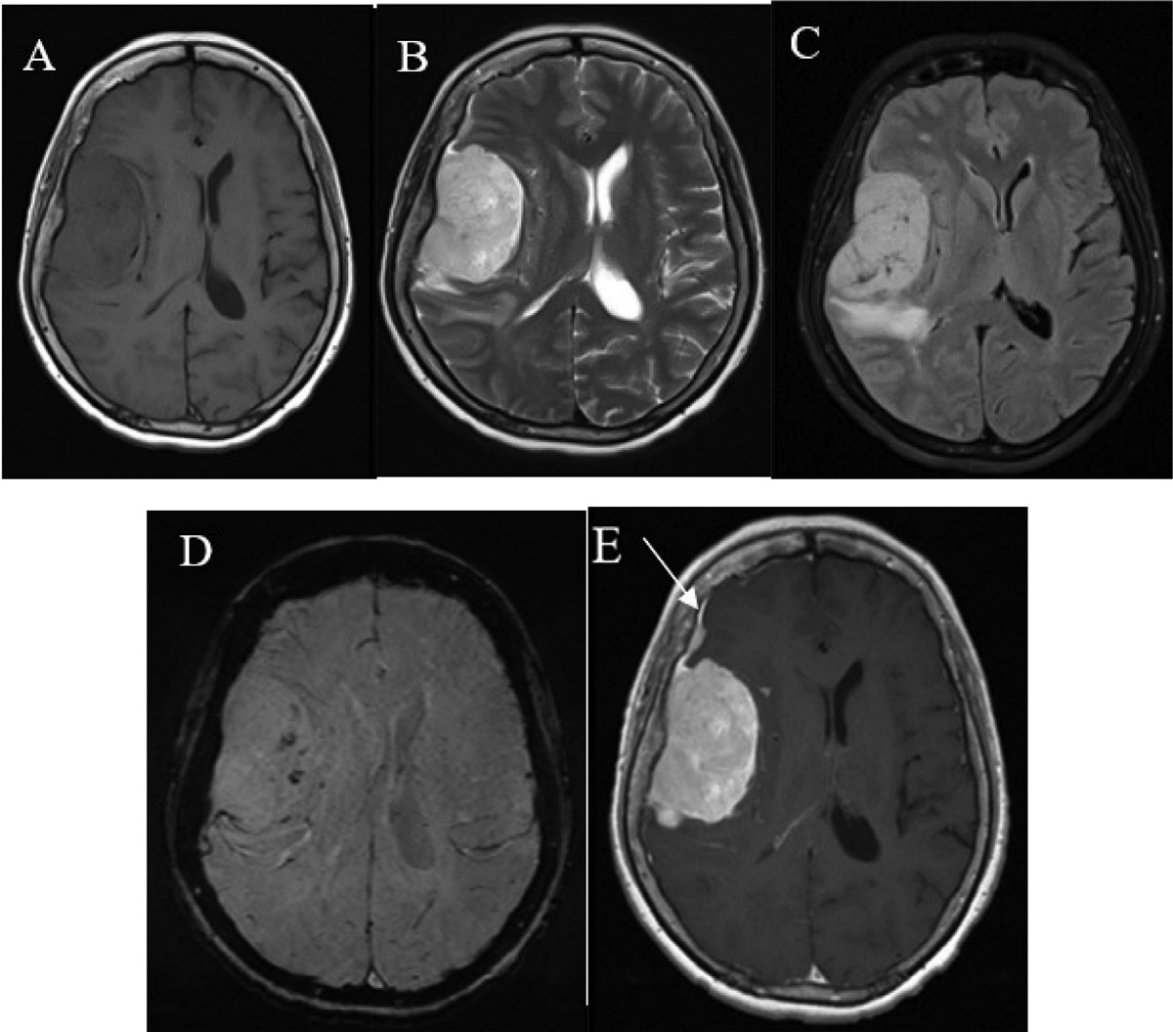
MRI showed hypointense mass in T1W1 (A), hyperintense frontotemporoparietal dextra region mass in T2W1(B), hyperintense mass in T2-FLAIR (C), blooming artifacts in SWI (D), T1+ Contrast showed enhanced mass, *dural tail sign* (white arrow) (E).
